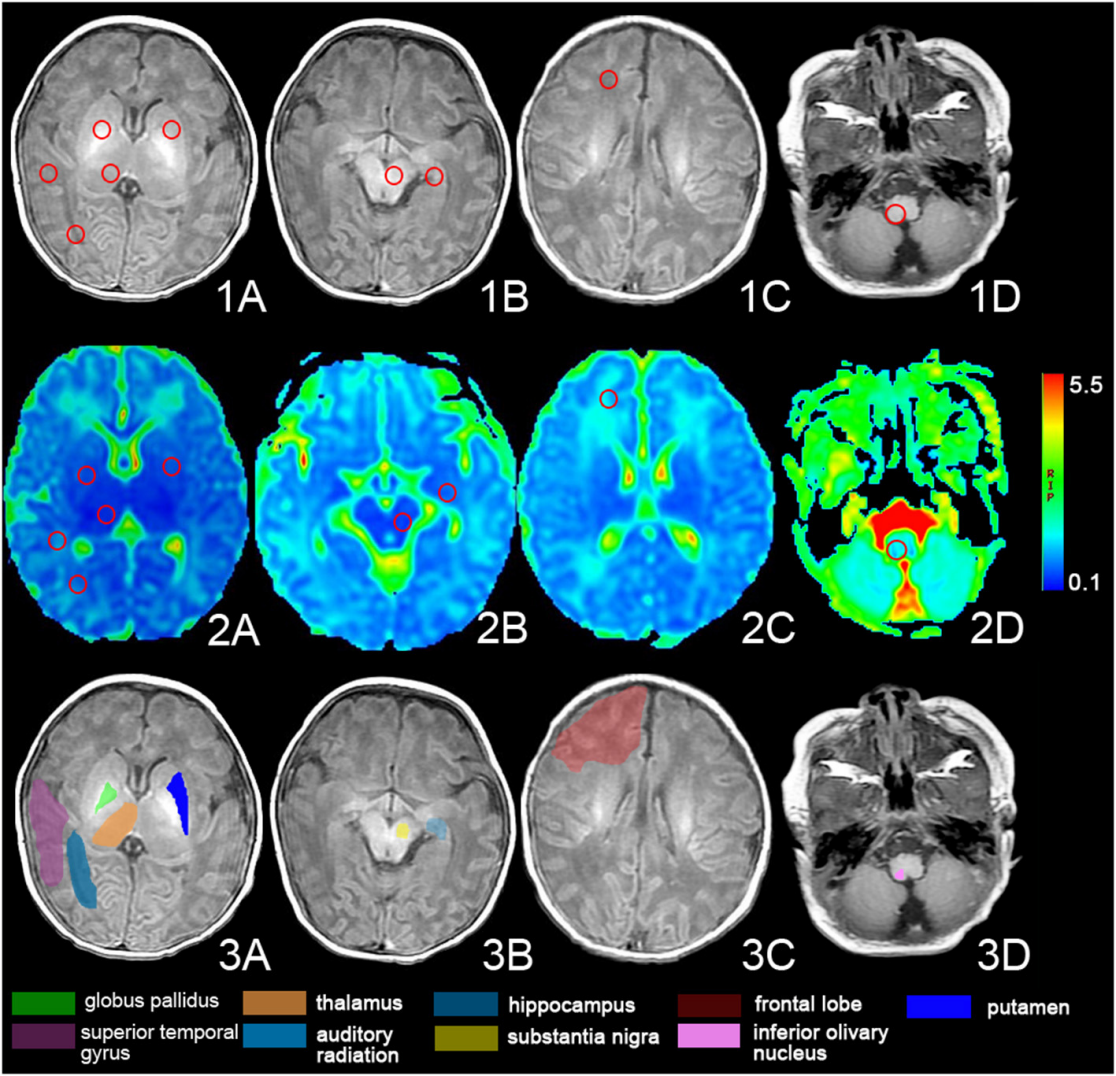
The regions of interest (ROIs) of the DKI. Images show the ROIs of T1-weighted images (1. A–D) and DKI images (2. A–D) and the anatomy of the ROIs (3. A–D). The ROI was set in bilateral globus pallidus, dorsal thalamus, frontal lobe, auditory radiation, superior temporal gyrus, substantia nigra, hippocampus, putamen, and inferior olivary nucleus.
